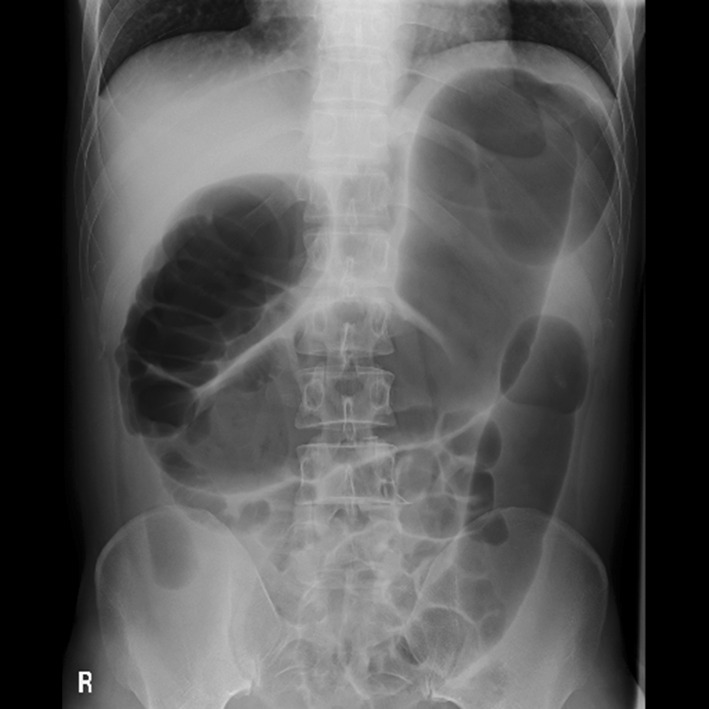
Abdominal X-ray after stoma closure. Large intestine was markedly dilatated
